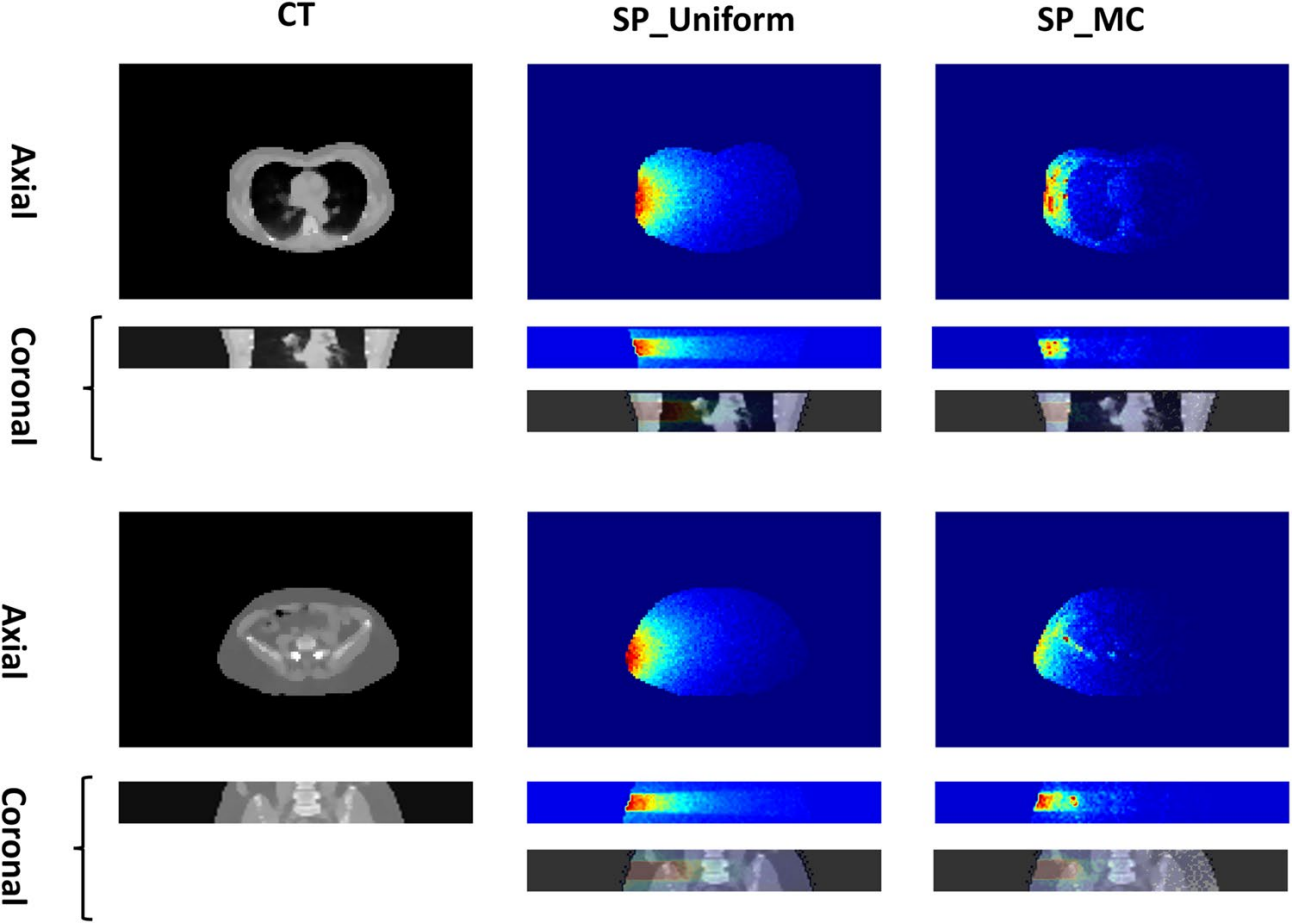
Examples of axial and coronal slices of CT, SP_Uniform, and SP_MC dose maps corresponding to a single source position/angle. In these cases, the X-ray tube is in the right lateral position
